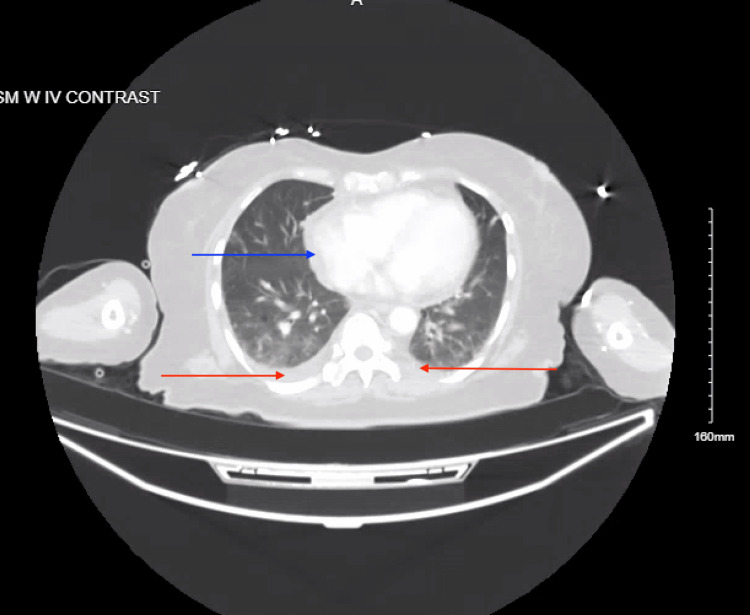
Axial section of a chest computed tomography with IV contrast demonstrating small bilateral pleural effusions (red arrows) and small pericardial effusion (blue arrow)
